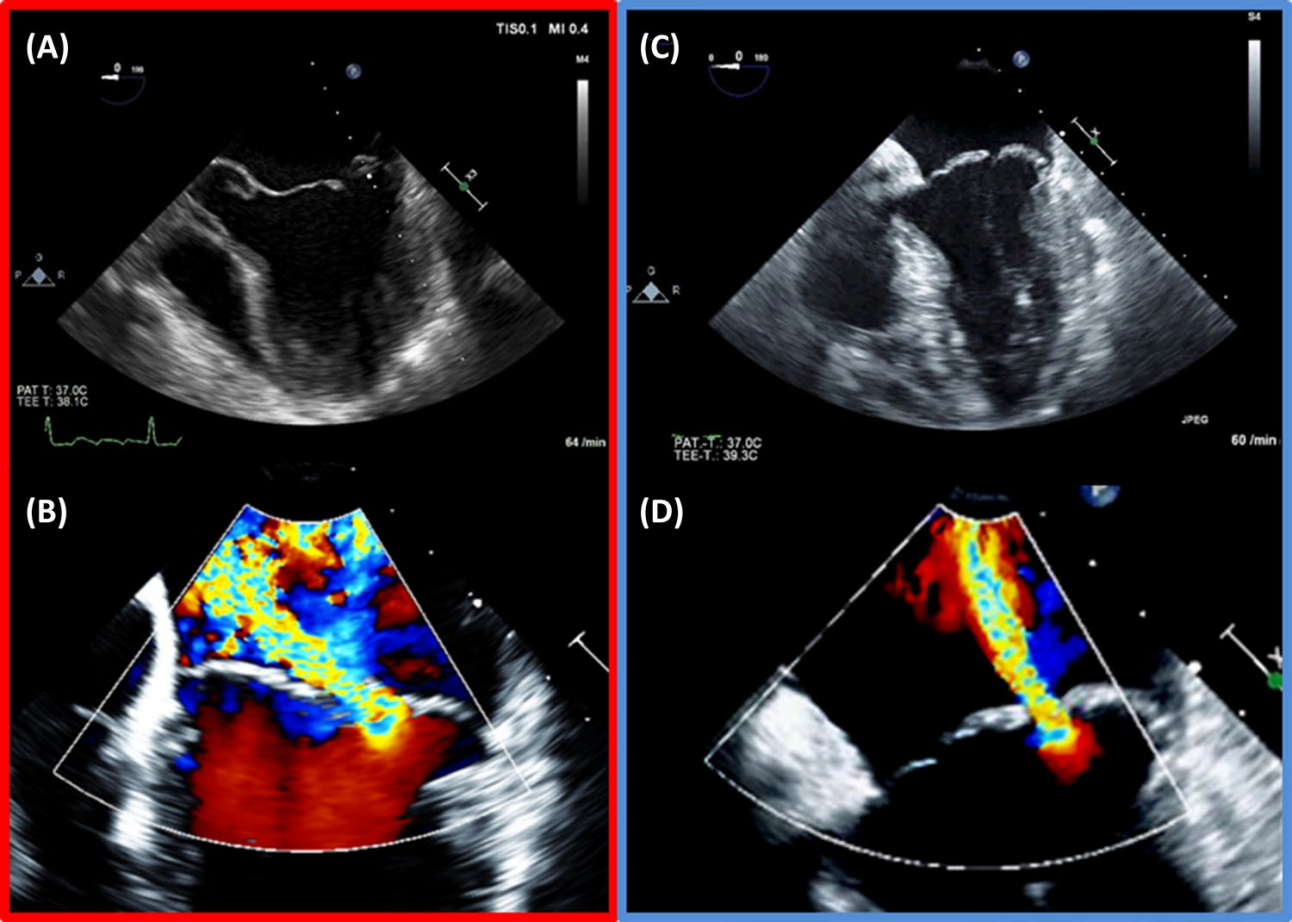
Echocardiographic images of the two different patterns of Barlow´s disease. Asymmetric type with the posterior leaflet predominantly prolapsing (a) and anteriorly directed regurgitant jet (b). Symmetric type with both leaflets prolapsing to the same height (c) and a central regurgitant jet (d)
